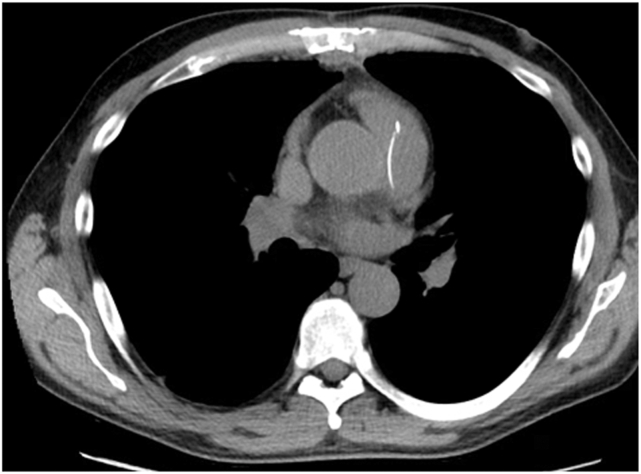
Postoperative CT scan, noncontrast. Axial image showing complete removal of calcium; calcium score is 0. *CT*, Computed tomography.
